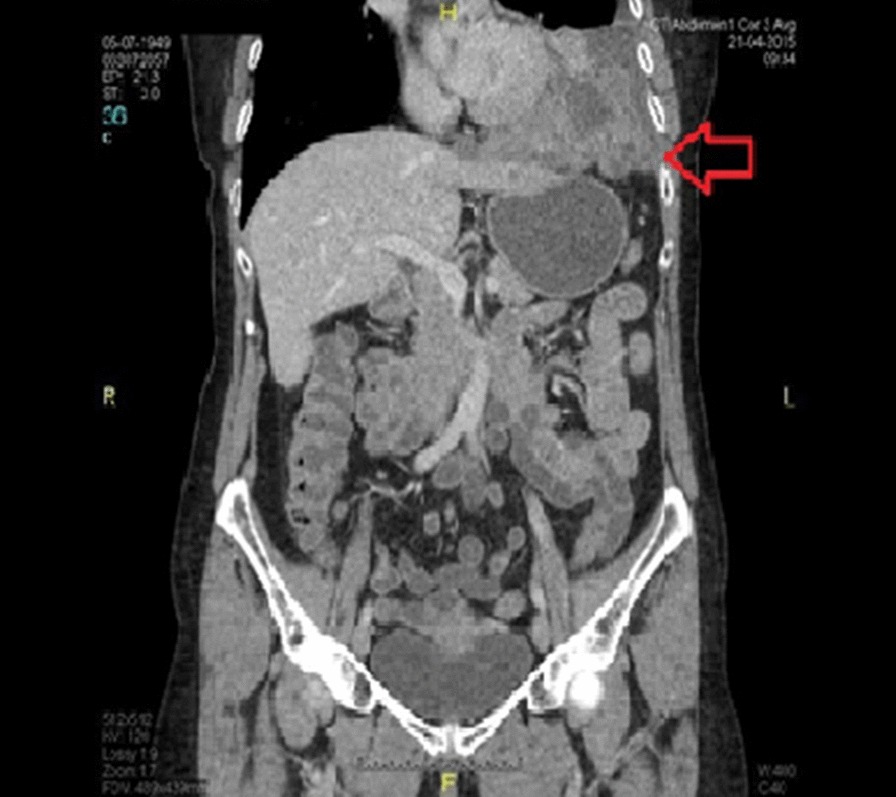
Preoperative computed tomography (CT) showing the lesion on the left diaphragm (red arrow)
